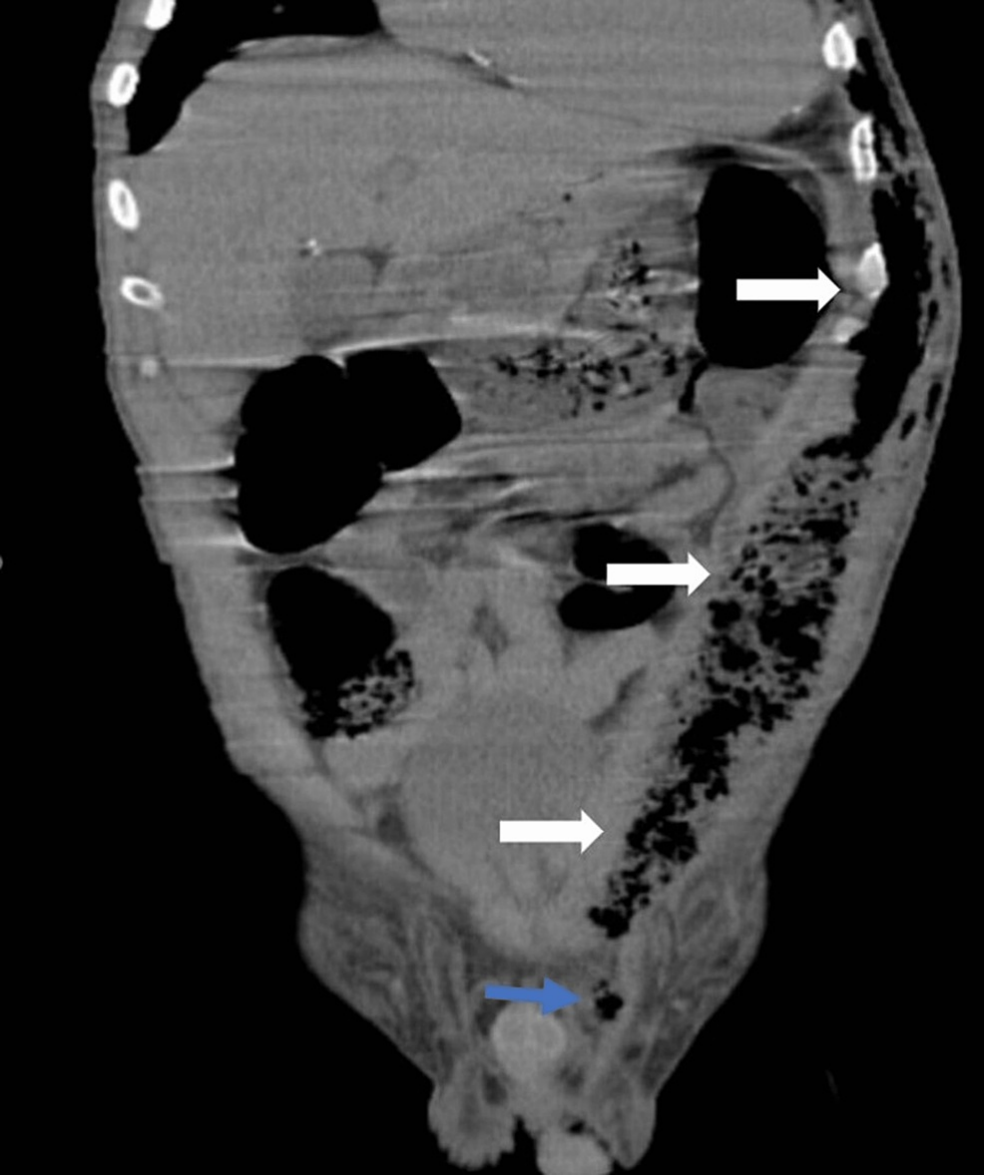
Coronal slice of thoraco-abdominopelvic CT: white arrows highlighting subcutaneous emphysema in the thoracic wall; blue arrow highlighting perineal abscess.
